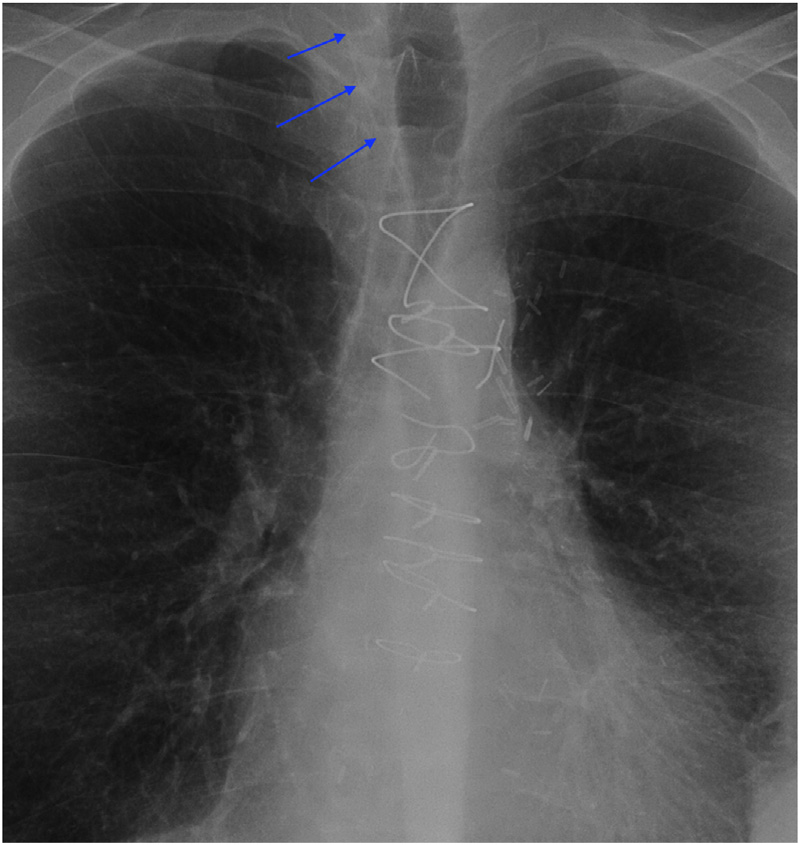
Chest X-Ray Demonstrating the TEPW Migration Into Ascending Aorta and Carotid Artery Chest x-ray 5 months after the operation showing retained temporary epicardial pacing wire coursing along the ascending aorta into the right common carotid artery, indicated by the blue arrows.
